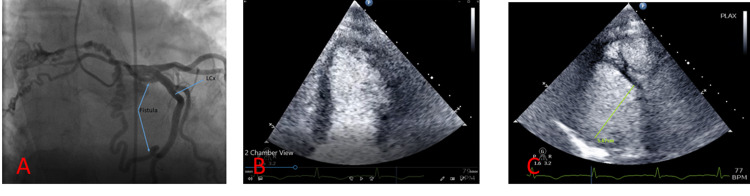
(A) Coronary angiography showing fistulous connections between the left circumflex (LCx) and the pulmonary artery in the absence of coronary artery disease. (B) Contrast echocardiography showing regional wall motion abnormality with severe hypokinesis of the apex and mid left ventricular segments with preservation of the basal segments. (C) Contrast echocardiogram demonstrating moderately dilated left ventricle (left ventricular internal diameter in diastole = 5.8 cm).
